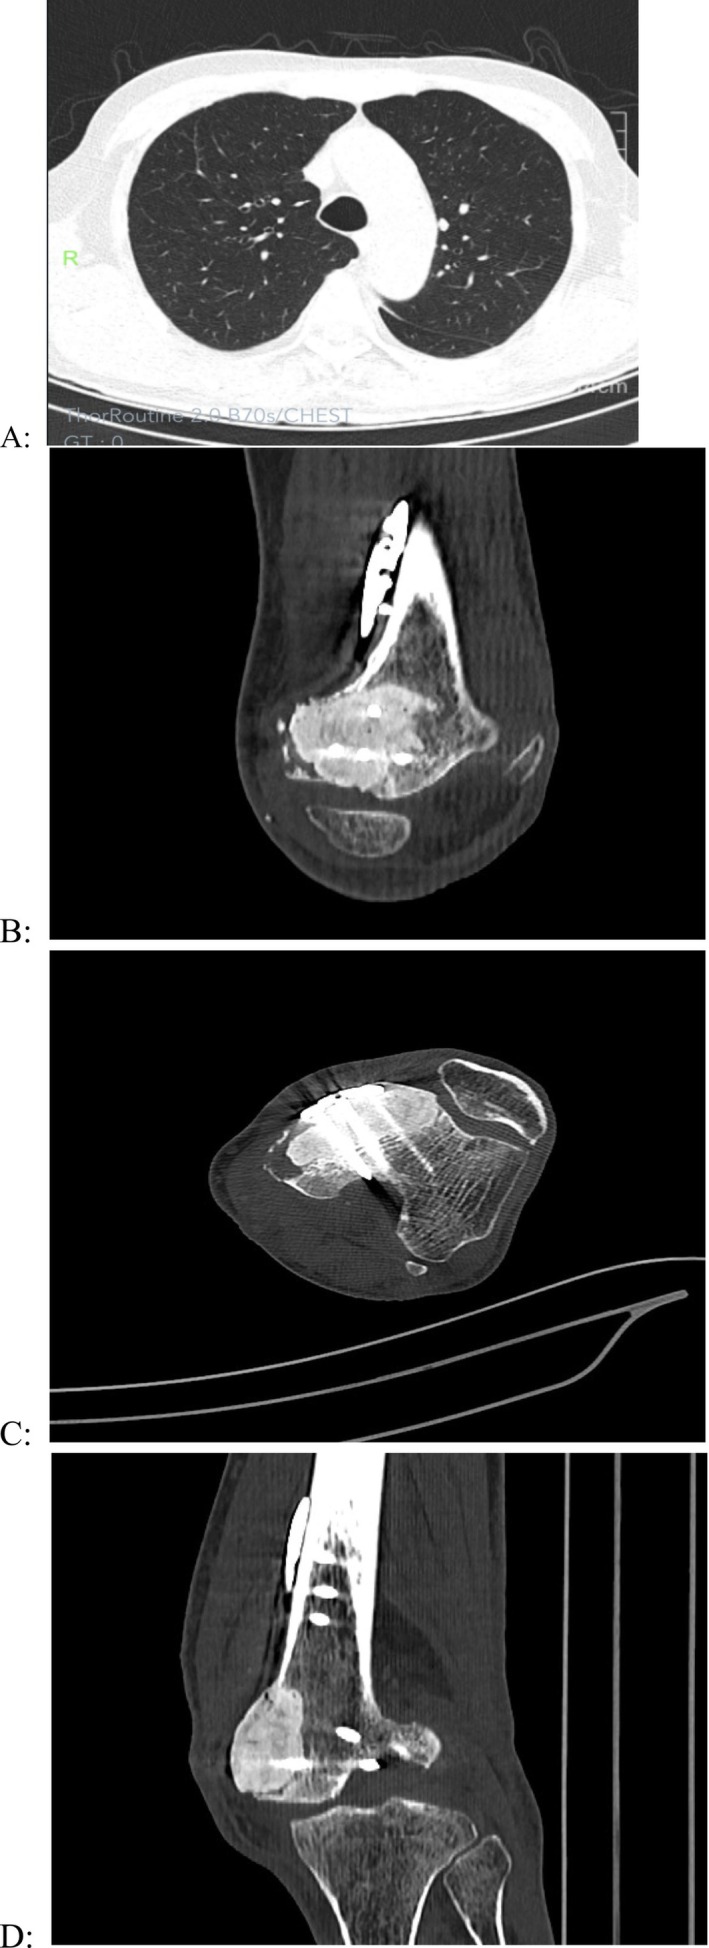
Post‐radiotherapy follow‐up imaging at 3 months: Chest CT (A) Unenhanced lung window shows clear bilateral bronchovascular bundles without abnormal course/distribution. Multiple tiny nodules are noted bilaterally. Left knee joint CT (B–D): Status post left femoral lesion resection, bone cement filling, and internal fixation. Internal fixation artifacts and patchy dense bone cement opacities are seen in the distal left femur. The medial cortex of the distal femur is thinned, discontinuous, with localized decreased bone density. Slight depression is present on the left medial femoral condylar articular surface, and angular/lip‐like changes are noted at the edges of left knee joint components.
